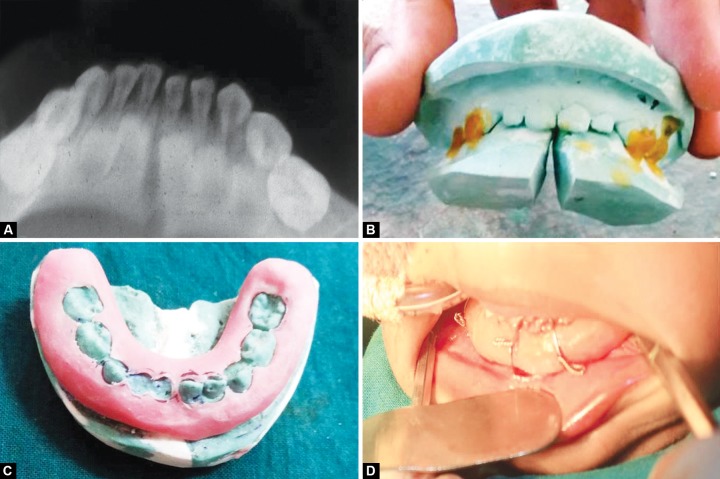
(A) Preoperative radiograph, (B) fabrication of MacLennan splint, (C) fabricated MacLennan splint, (D) MacLennan splint with circummandibular wiring
